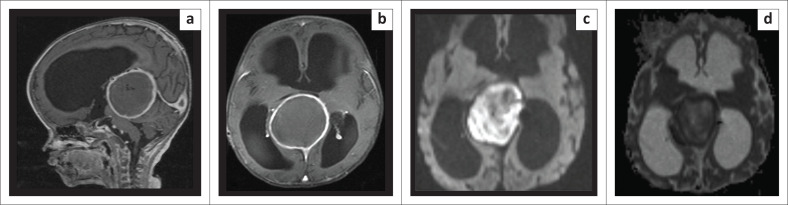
Follow-up MRI at 1-month post-procedure demonstrating the chemical abscess: (a) T1FS post-contrast mid-sagittal, (b) T1-fat-saturated post-contrast axial, (c) Diffusion-weighted imaging axial at b 1000 s/mm^2^ and (d) Apparent diffusion coefficient axial at the same level as 3c; showing a mild reduction in the size of the aneurysmal sac, persistent hydrocephalus, thick peripheral mural enhancement and marked restricted diffusion.
